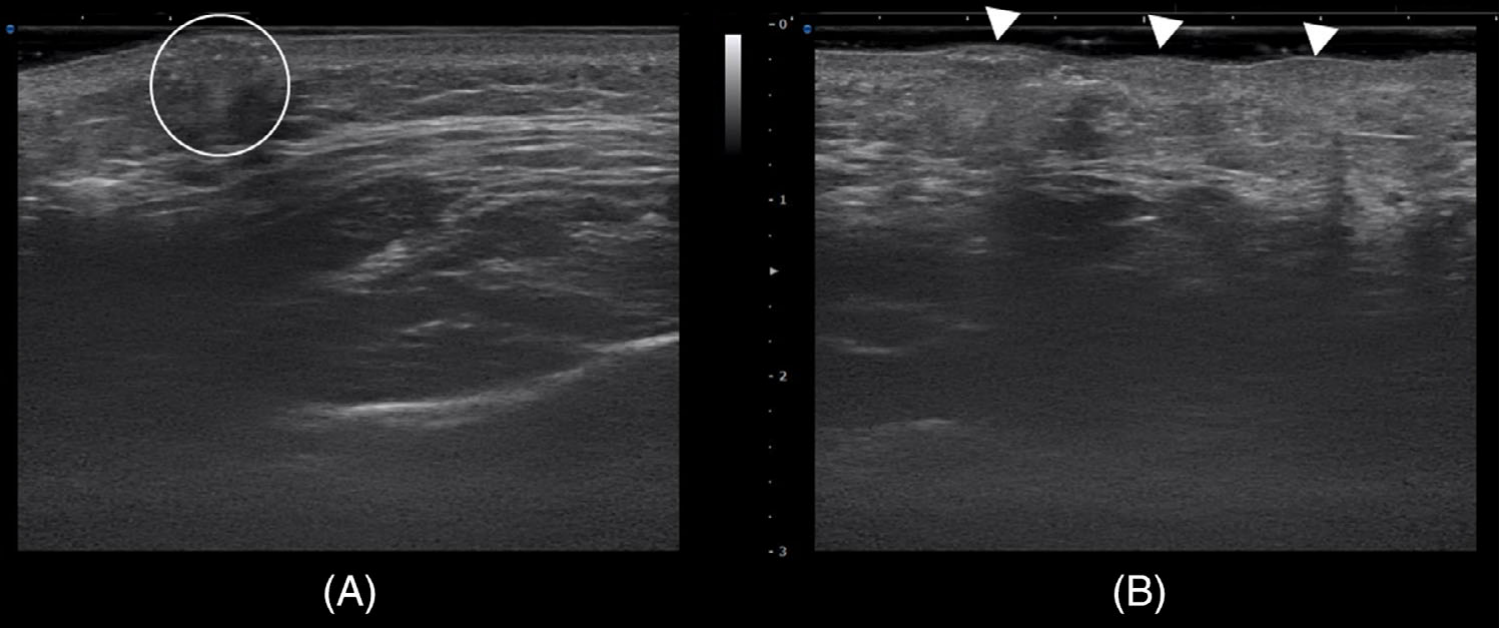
The figure depicts ultrasonography results, where posterior shadowing (A, circled) is observed. The polynucleotide was injected at a distance of 1 cm (B, white arrows).
